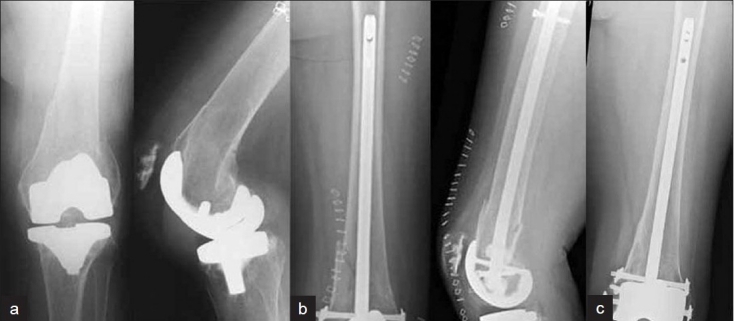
(a) Anteroposterior and lateral radiographs of a 60-year-old male with total knee arthroplasty who suffered a periprosthetic distal femur fracture. (b) Closed reduction and intramedullary stabilization was done using SCN (c) followup X-rays shows bony union
